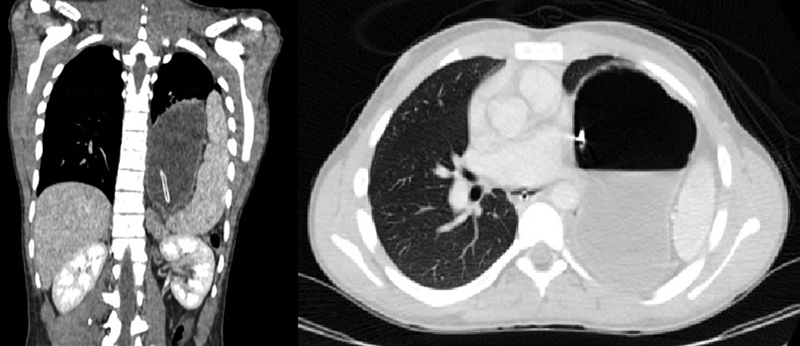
Coronal and axial view of contrast-enhanced computed tomography.
